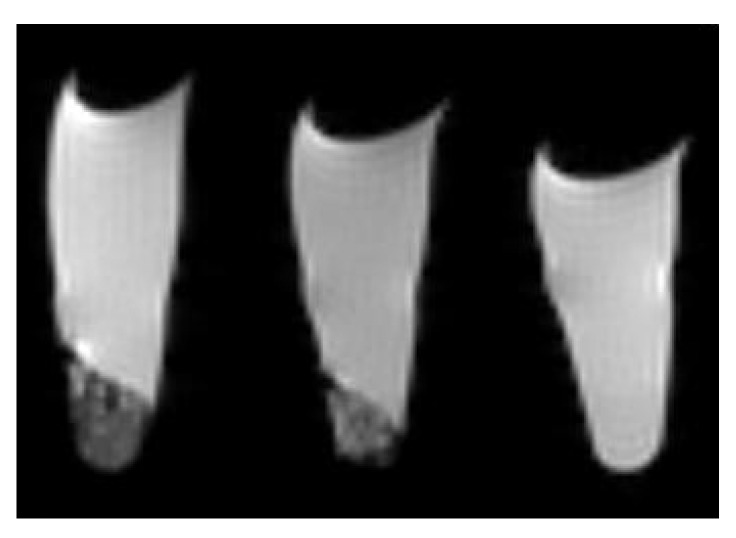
T_2_ Weighted MRI image (9.4T) of HT-29 cells incubated with no MSN (right), 300 µg MSN (4) (center), and 300 µg MSN-RGD (4a) (left).
